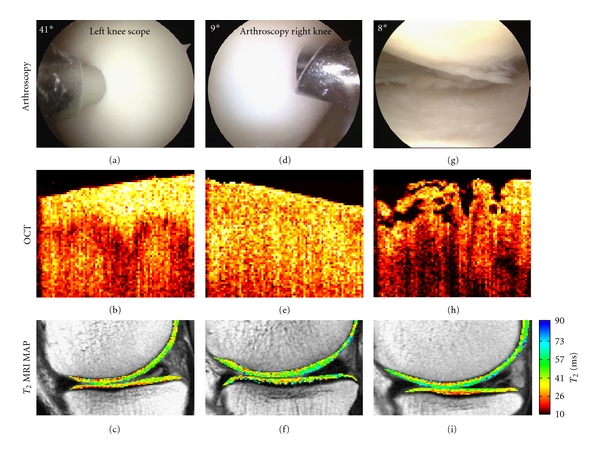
Representative images obtained during arthroscopy, optical coherence tomography (OCT), and magnetic resonance imaging (MRI) *T*
